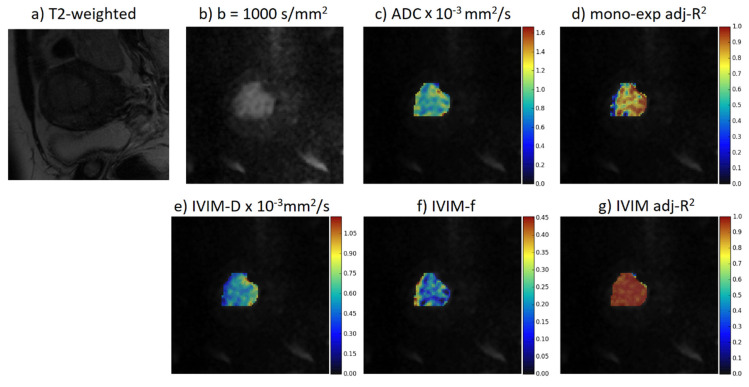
From top left to bottom right: A T2-weighted MRI, DWI acquired at b = 1000 s/mm^2^, and parametric maps for the ADC, adj-R^2^ when the diffusion signals were fitted by the mono-exponential model, D, f, and adj-R^2^ when the diffusion signals were fitted by the bi-exponential model. ADC: apparent diffusion coefficient; adj-R^2^: adjusted R-squared; D: diffusion coefficient; f: micro-perfusion fraction.
